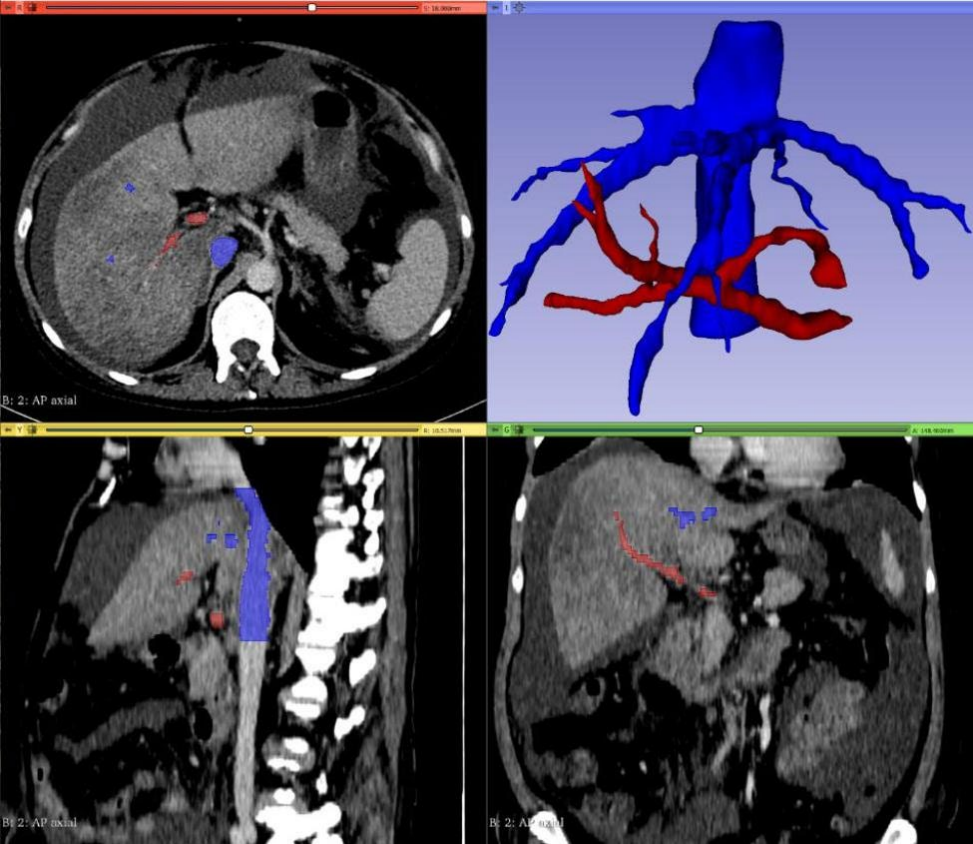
Segmentation Process A screen capture of the segmentation software 3D Slicer shows a model being created from a CT scan. On the top left, bottom right, and bottom left, different views of the patient’s imaging are displayed with bright colors overlaying the anatomy that has been selected from the background CT (red = portal venous, blue = hepatic/systemic venous). On the top right the resulting 3D geometry is displayed
